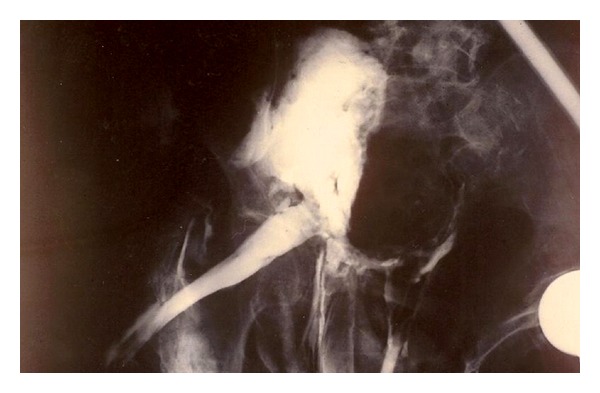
Plain anteroposterior radiograph showing the pelvis 7 minutes after per rectum administration of gastrografin. Gastrografin is escaping from the pelvic cavity through a fistula to the thigh.
